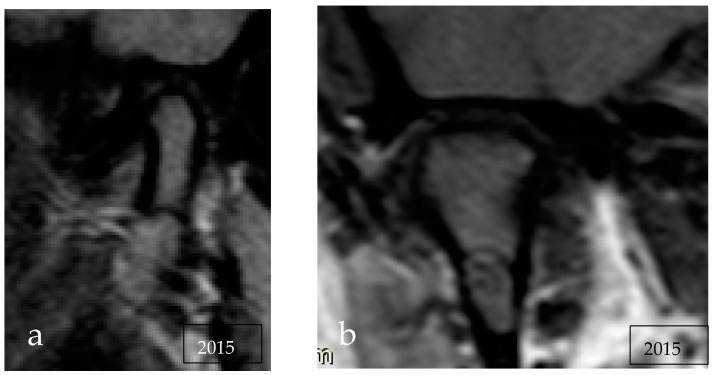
MRI scans showing an intraosseous lesion isointense to bone marrow and with a hypointense rib: (a) sagittal projection, (b) coronal projection.
